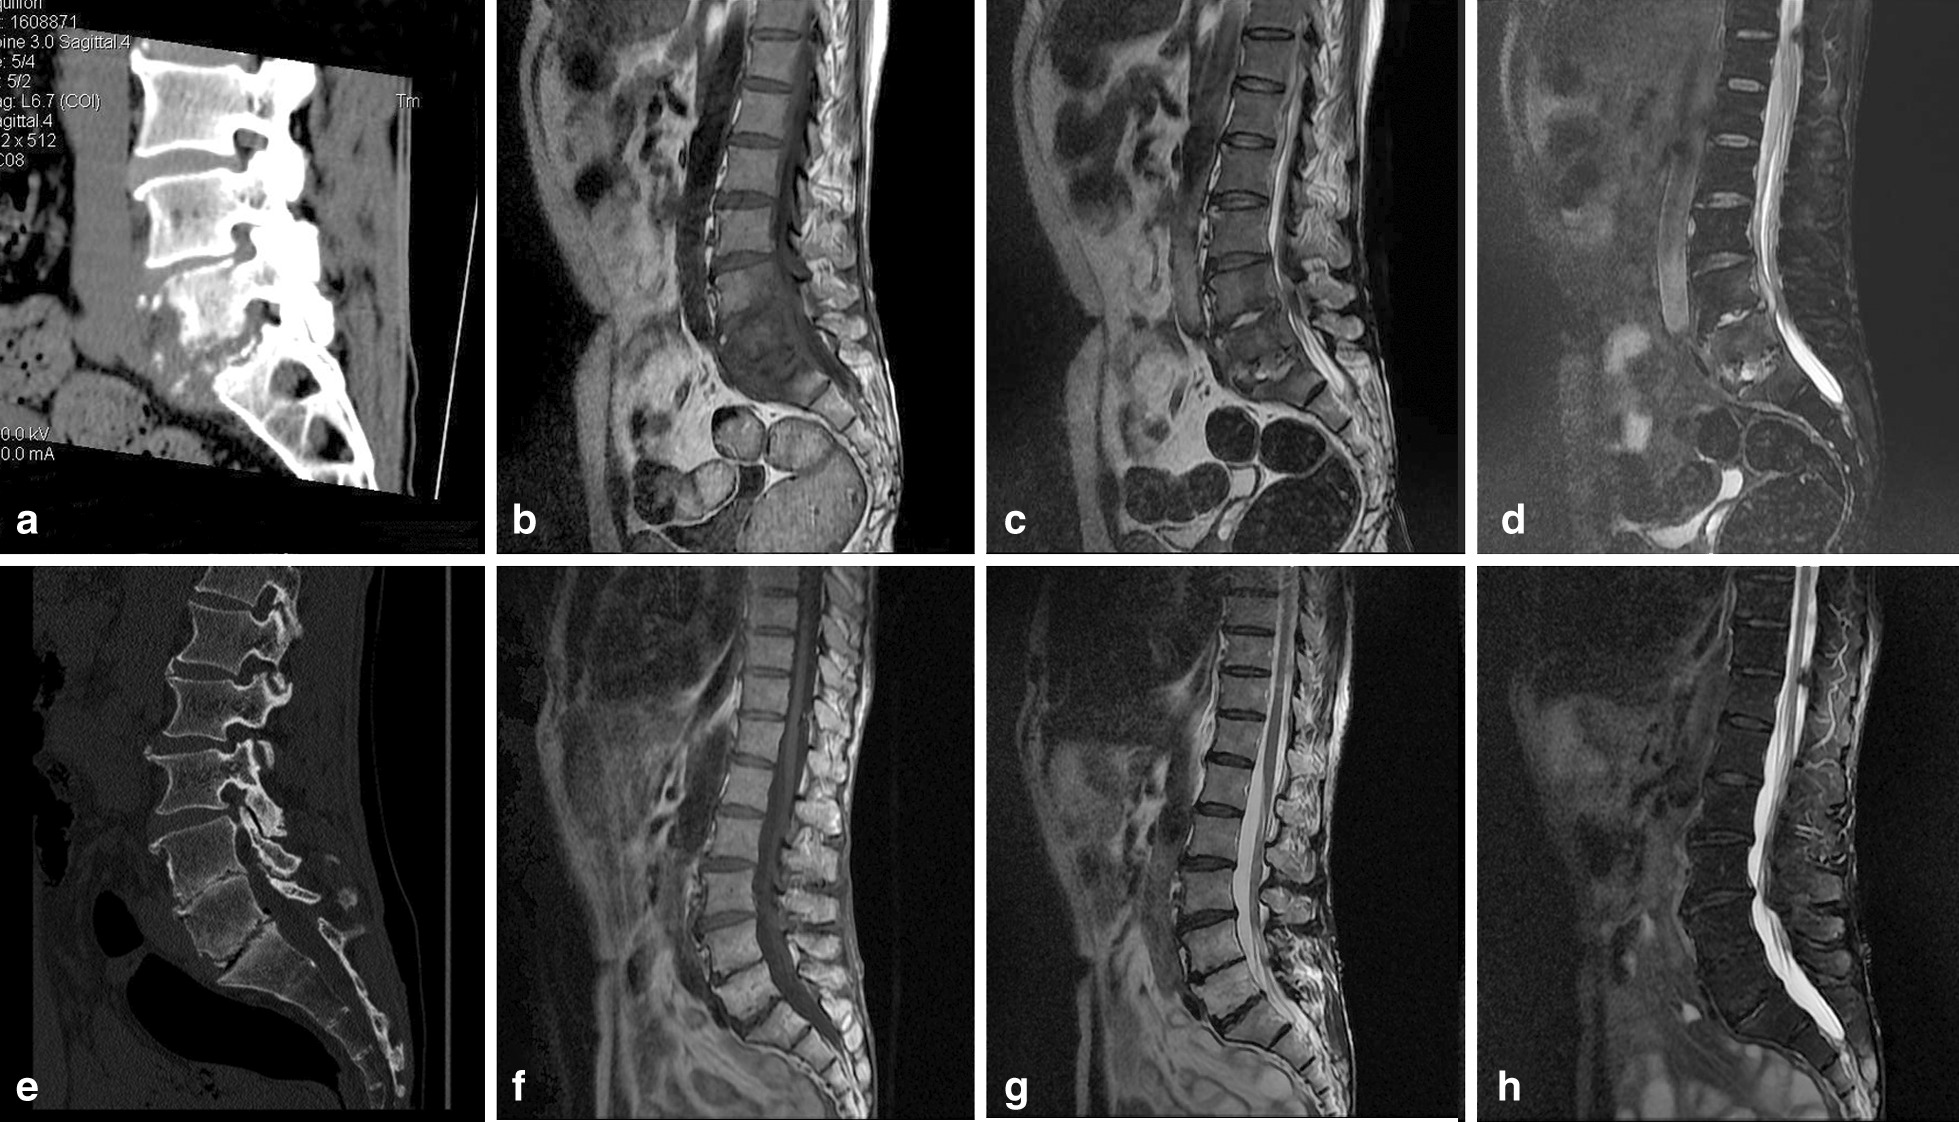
Dynamic changes in the lumbar vertebra on computed tomography (CT) scan. Bone destruction at the upper and lower edges of the lumbar vertebra and the superior border of the sacrum were observed in Dec 2010 (a, b, c, d). No obvious bone destruction and disappearance of soft tissue swelling in July 2016 (e, f, g, h)
